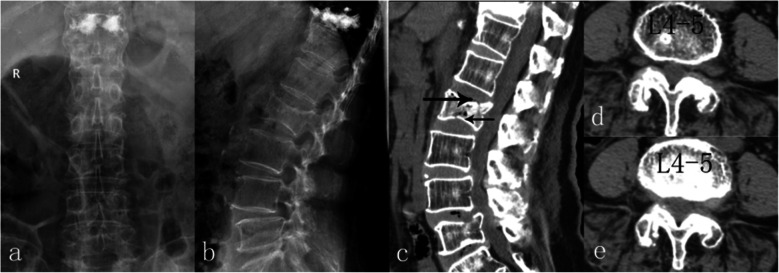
DR 8 weeks postoperatively revealed shortened height of L2 (a-b). CT examination detected a fresh comminuted fracture of L2 vertebral body; the irregular sharp fracture lines (arrow) indicated acute or subacute fracture (c). Transverse images at L4–5 level (d-e) revealed no reherniation of disc
